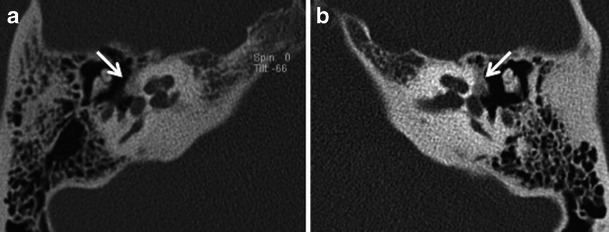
Axial HRCT images of the *right* (a) and *left* (b) temporal bone in an adult patient with bilateral CHL. Hypodense demineralised plaques (*arrows*) are noted in bilateral fissula ante fenestram regions in keeping with bilateral fenestral otosclerosis
